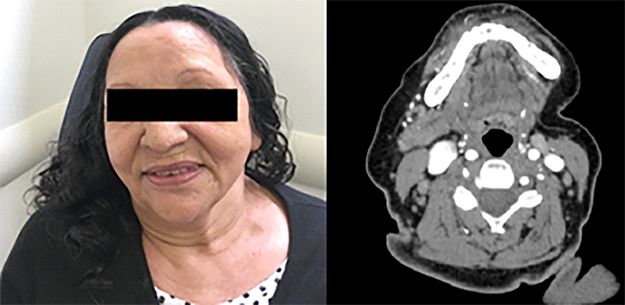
Absence of cervical tumorations 72 months after admission. CT scan maintained resolution of left submandibular gland tumor 75 months after remission.
